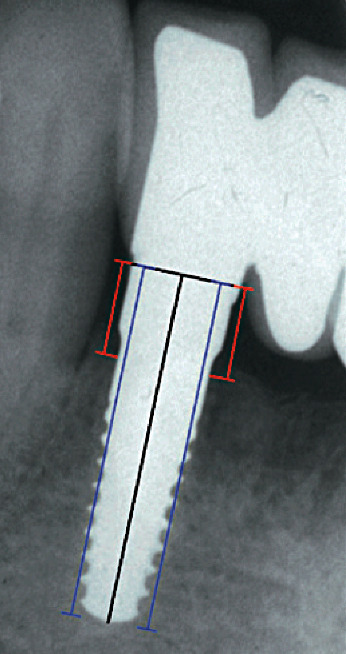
120 months after surgery: radiographic check of a zirconia dental implant positioned in 4.2. Assessment of the distance from the shoulder to the first bone-to-implant contact on digitalised radiographs (red lines). The blue lines refer to implant length.
